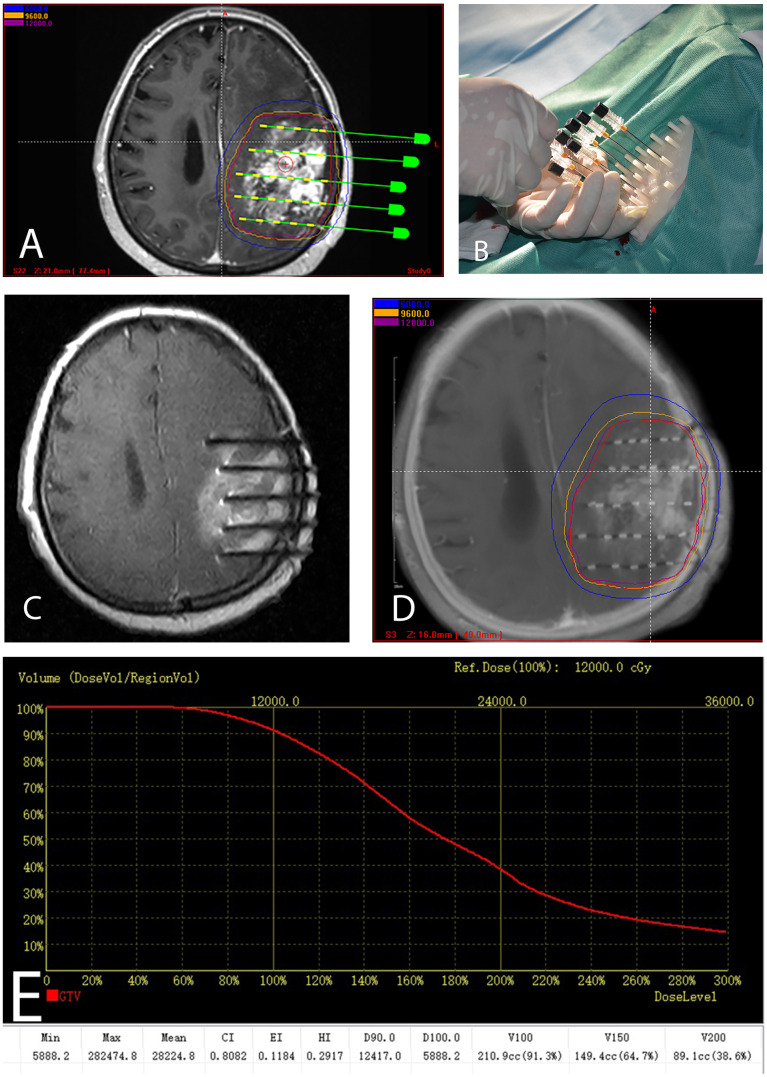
A patient with recurrent glioblastoma after surgery, radiotherapy, and temozolomide chemotherapy underwent ¹²^5^I seed implantation assisted by a 3D-printed template. (A) Pre-operative treatment plan. (B) Blunt-tip needles were inserted into the tumor through the puncturing template. (C) The positions of needles were visualized on axial MR images. (D) Post-operative dosimetry verification based on post-operative CT/MR fusion images. (E) Postoperative dose volume histogram (D90 = 124.2 Gy).
